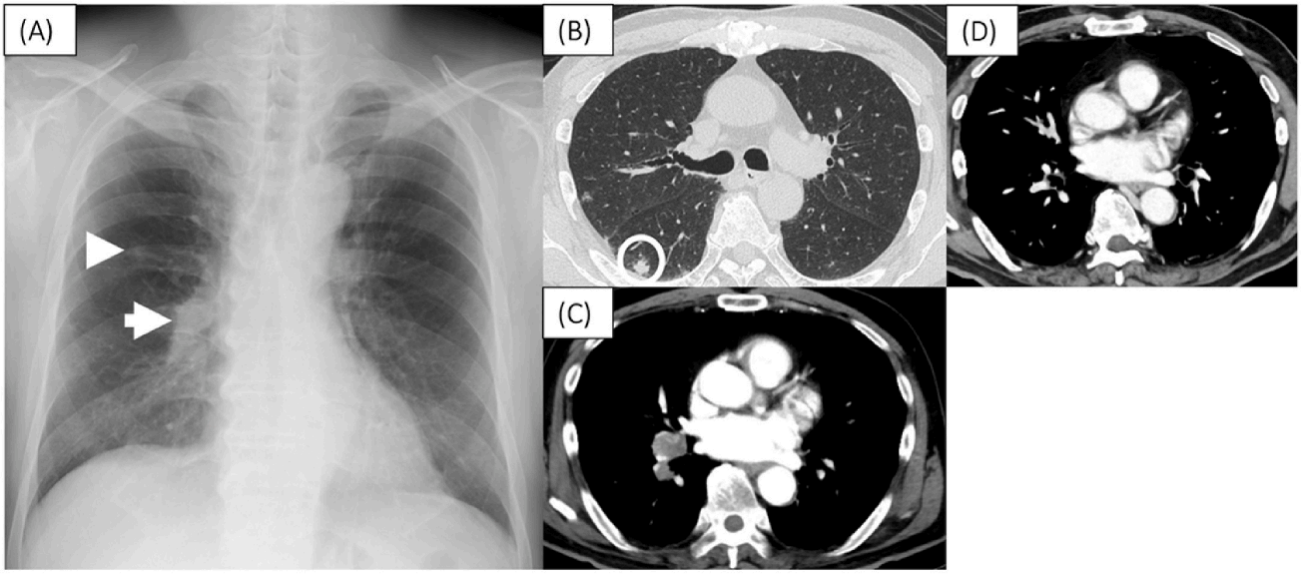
Chest X-ray film on admission showed tumor shadows in the right middle lung field (arrowhead) and right hilar hilum swelling (arrow) (A). Chest CT on admission. CT showed an 11mm mass in the right lower lobe (circle) (B). Contrast-enhanced CT showed multiple right hilar hilum lymph swelling (C). CT after 6 courses of durvalumab showed marked reduction of hilar lymph nodes (D).
